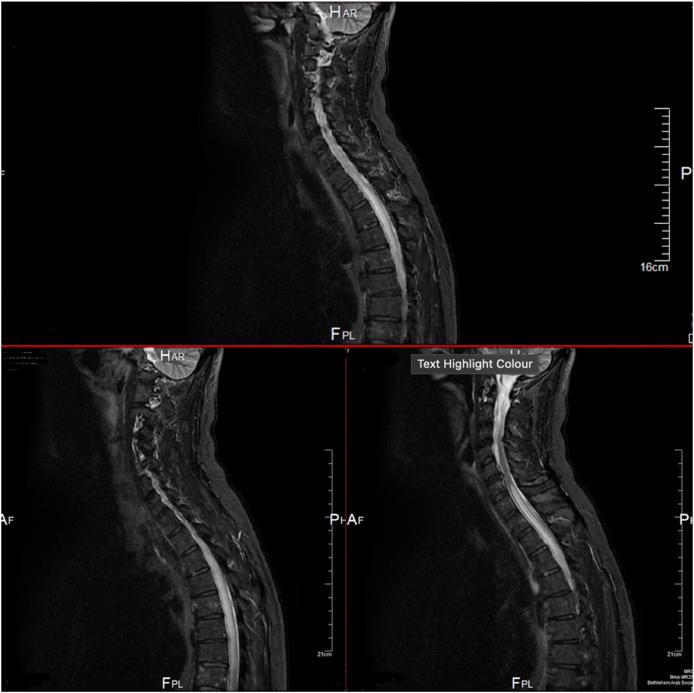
An MRI performed of 5^th^ November 2020 showing abnormal signal intensity within the thoracic cord extending from the mid T6 vertebral body level (superiorly) to the T11-T12 disc level inferiorly.
